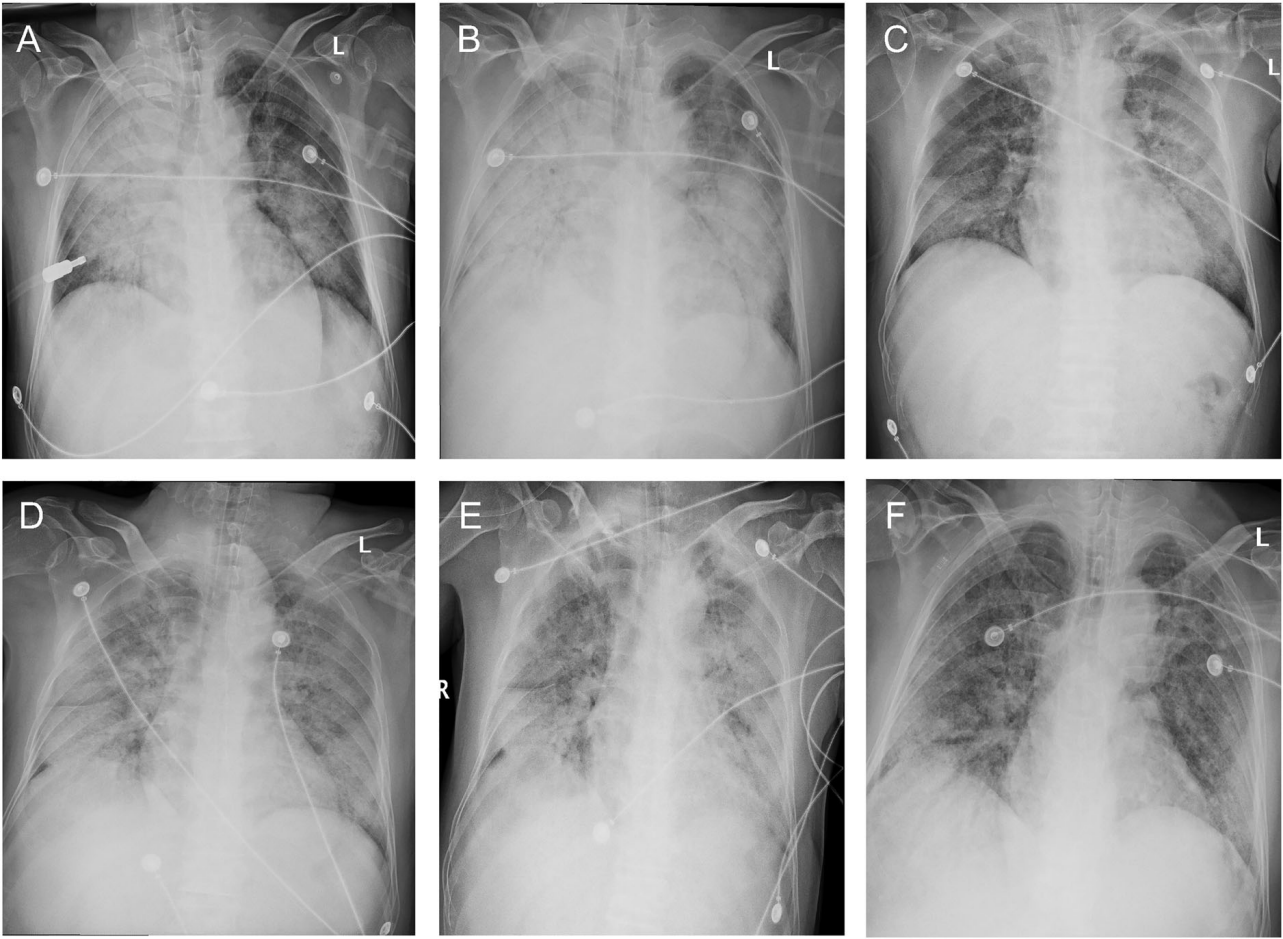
Chest radiographs during the clinical course. Bilateral ground-glass opacity and consolidation were observed on day 9 (Dec 26, Panel A) and worsened on day 11 (Dec 28, Panel B). Bilateral lung involvement was slightly relieved on day 14 (Dec 31, Panel C) after methylprednisolone was administrated on day 11. Bilateral ground-glass opacity and consolidation continued till day 16 (Jan 2, Panel D) and day 18 (Jan 4, Panel E) and relieved slightly on day 20 (Jan 6, Panel F).
